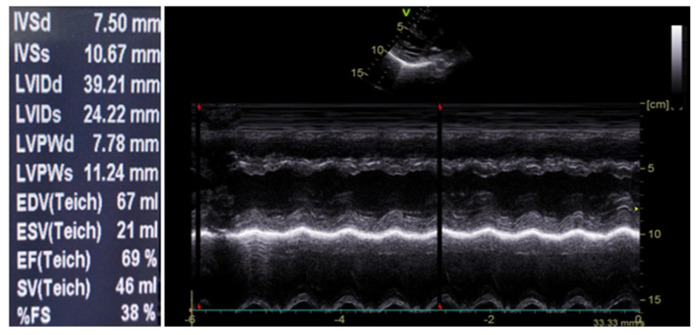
Cardiac echocardiography on Day 2.
